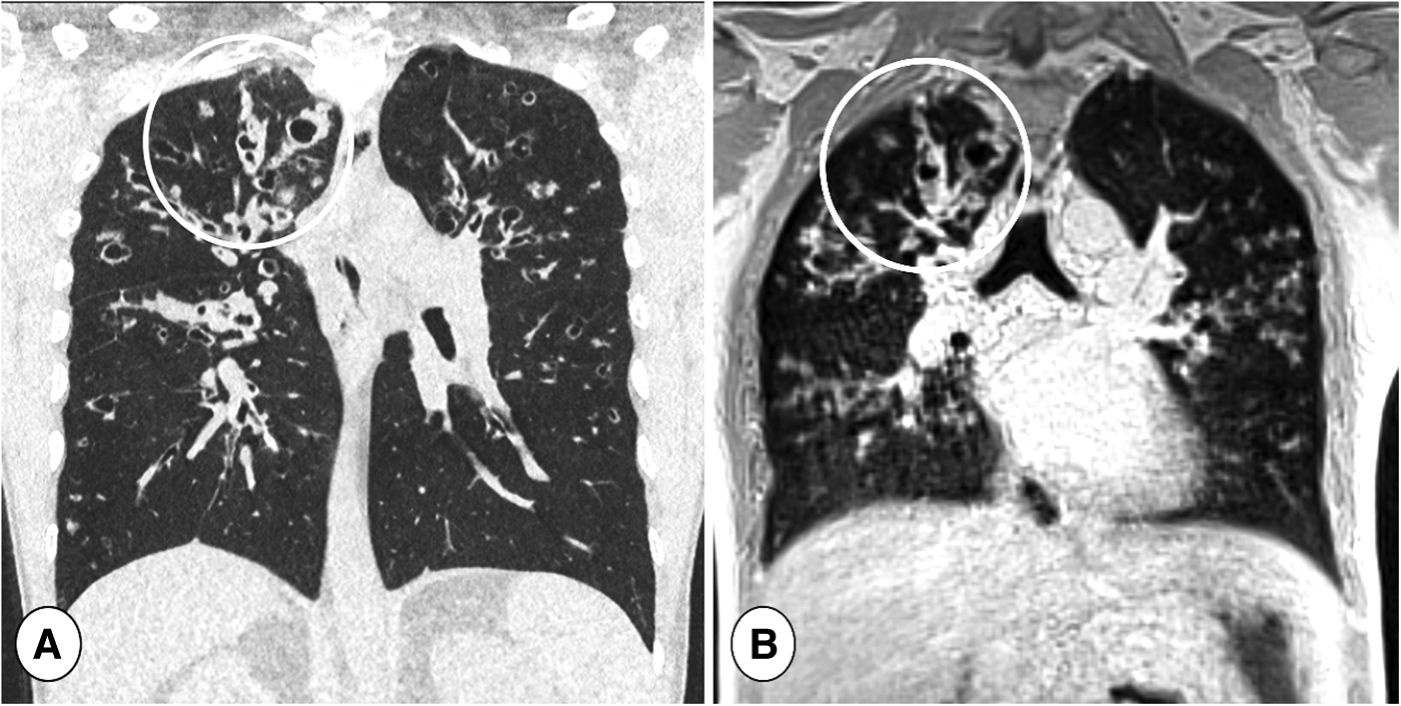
Coronal reformatted CT (A) and coronal ultra-short TE (UTE) MR scan (B) in a cystic fibrosis patient during respiratory tract exacerbation. Note the high definition of lung abnormalities in both CT and MRI in the right lung (circles).
